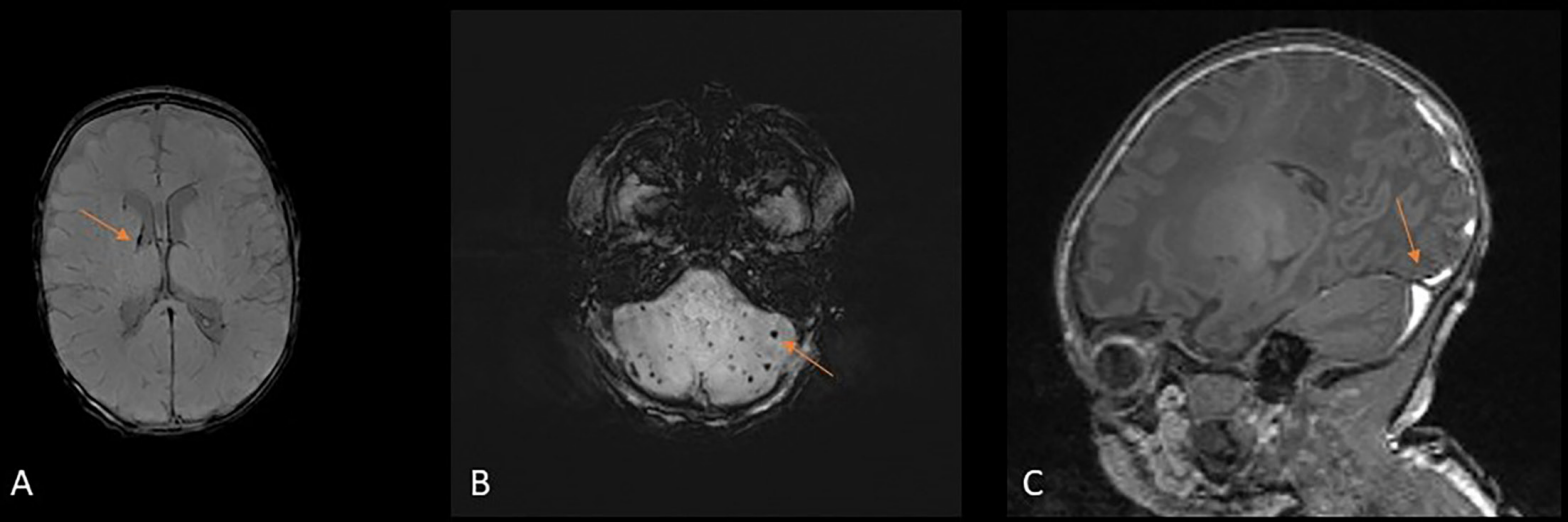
Intracranial haemorrhage. (A) susceptibility-weighted imaging (SWI) of infant aged 30^+3^ weeks, scanned at 40^+2^ weeks, showing residual germinal matrix haemorrhage-intraventricular haemorrhage. (B) SWI of infant aged 30^+0^ weeks, scanned at 40^+4^ weeks, showing multiple punctate cerebellar haemorrhages. (C) T1-weighted image of infant aged 39^+2^ weeks, scanned at 40^+3^ weeks, showing subdural haemorrhage.
